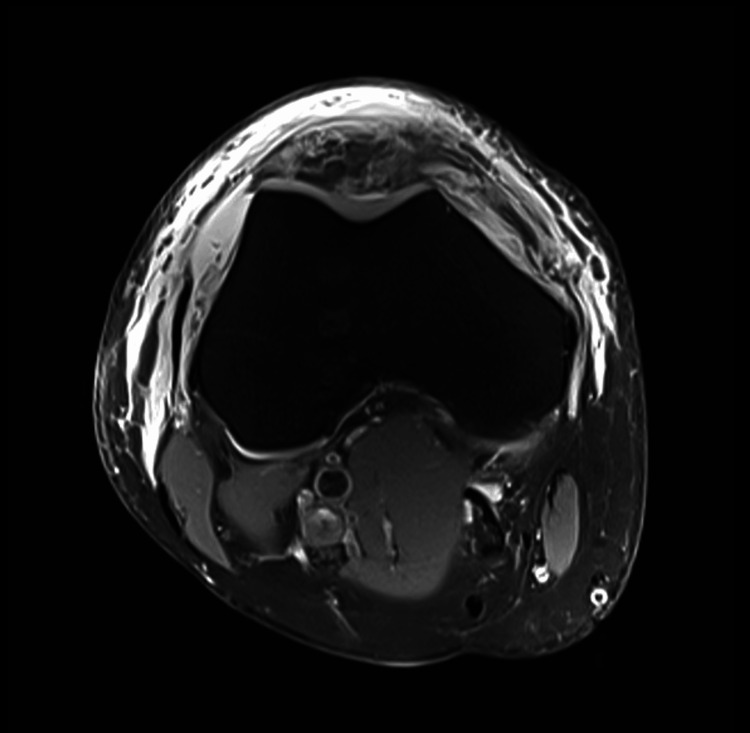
Axial MRI demonstrating associated extensor mechanism injury. Axial T2-weighted fat-suppressed MRI demonstrating associated soft tissue injury with edema and disruption of the lateral patellofemoral retinaculum and surrounding extensor mechanism structures.
